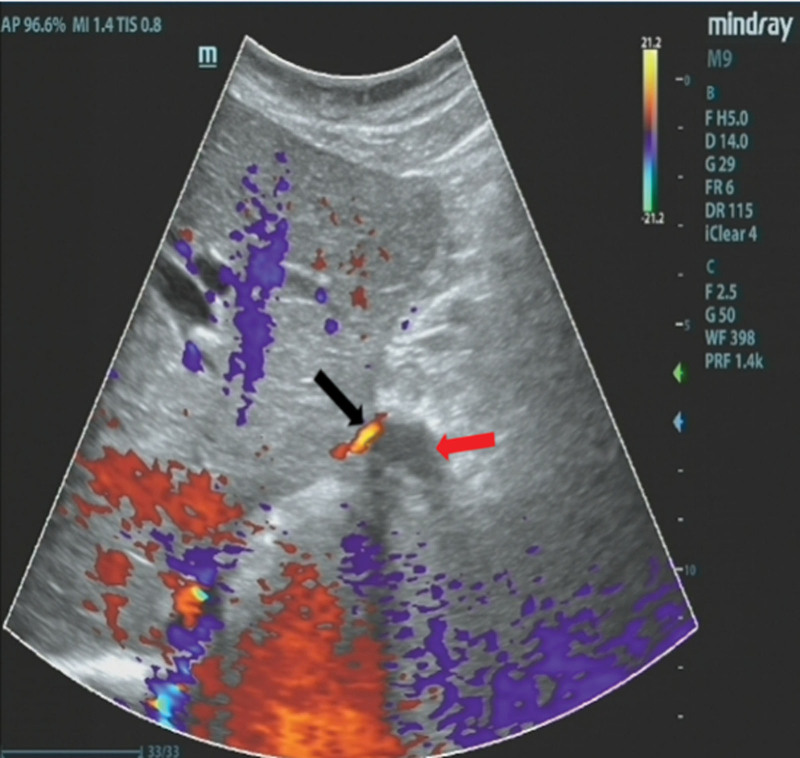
2D ultrasonography combined with Doppler ultrasonography showed blood flow (Black arrow) in front of the subdiaphragmatic fluid dark area (red arrow).
